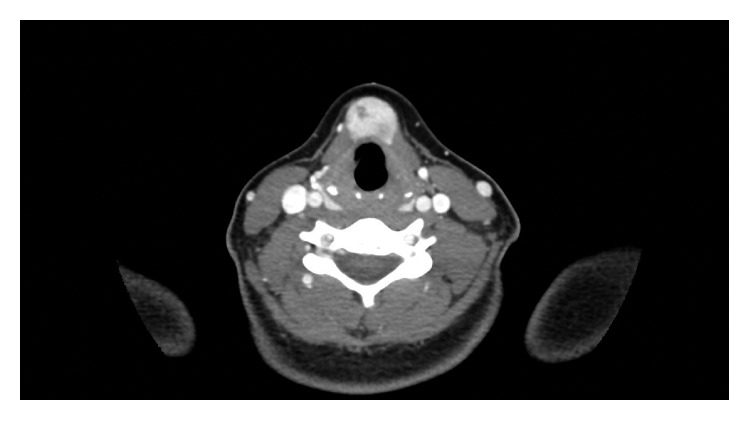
CT neck at the level of vertebra C4 showing a 1.6 × 2.0 × 2.9 cm, enhancing, elliptically shaped mass located in the midline, within the anterior soft tissues of the neck.
